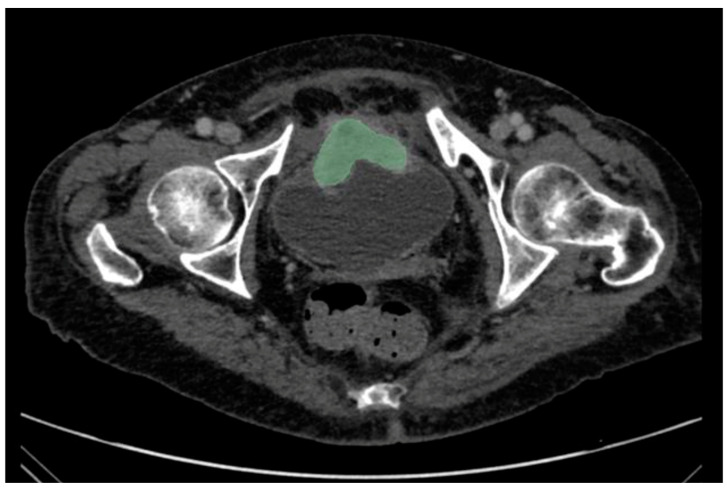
Manual segmentation was performed using 3D Slicer v. 5.0.3 software of a neuroendocrine bladder lesion in an 81-year-old subject on a contrast-enhanced CT scan.
